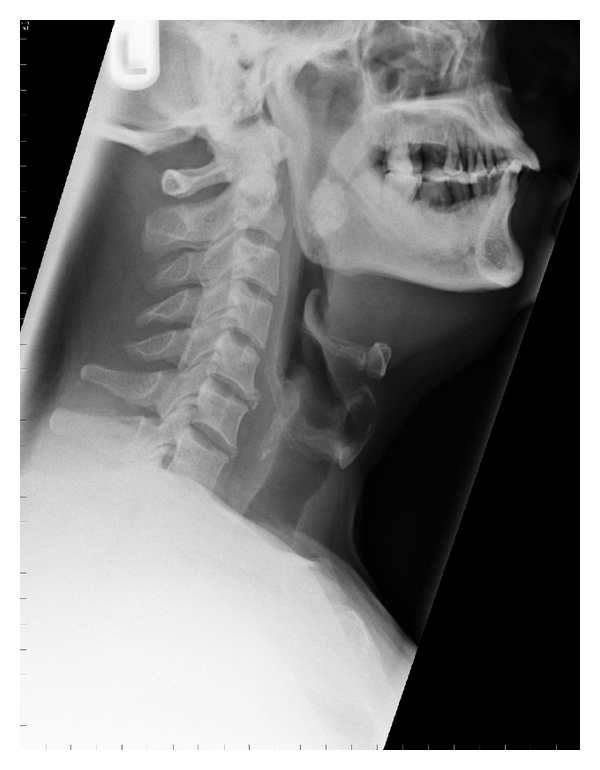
Lateral neck radiograph demonstrating a large tonsillolith.
